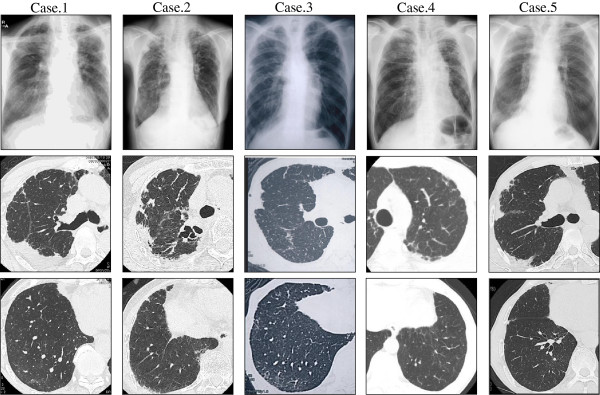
Chest X-ray and Chest CT. Chest radiographs in all cases showed marked apical pleural thickening. High-resolution CT (HRCT) showed upper lobe volume loss, architectural distortion, traction bronchiectasis, and reticular abnormalities. There was no honeycombing.
